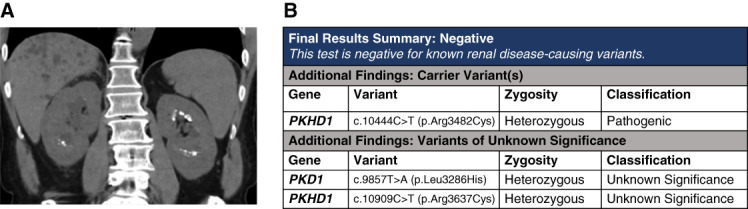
Imaging and sequencing results of case 3. (A) Axial CT imaging showing small, diffuse liver cysts, a few kidney cysts, and medullary nephrocalcinosis. (B) Sequencing result showing a carrier variant in *PKHD1* and VUSs in *PKD1* and *PKHD1*.
